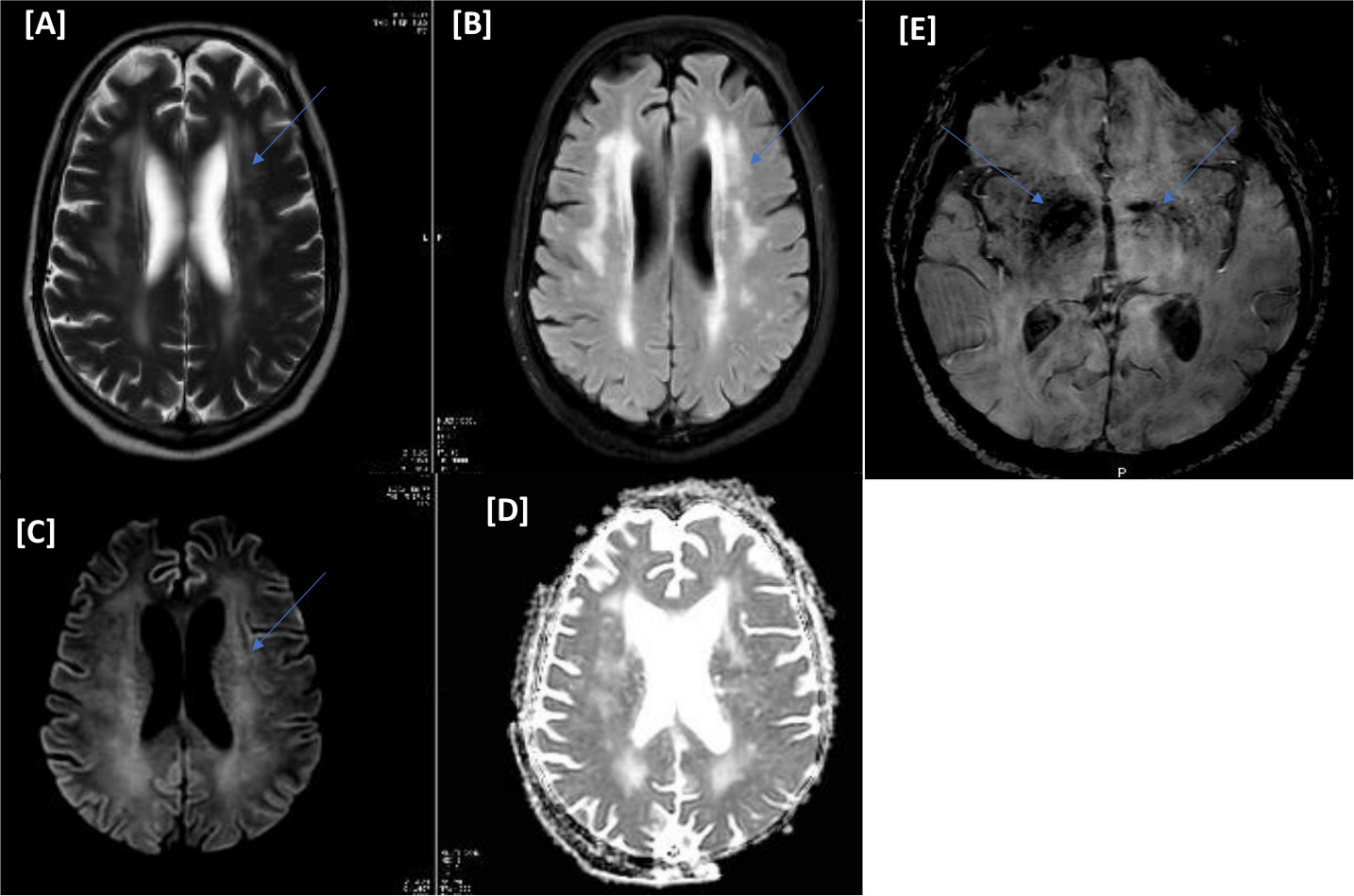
(Case I) [A–E images] Magnetic resonance imaging of the head. Diffuse periventricular white matter hyperintense signals on T2/FLAIR (A and B). No corresponding diffusion restriction on diffusion-weighted imaging b1000/apparent diffusion coefficient (C and D). Bilateral basal ganglia small storm susceptibility appearance on susceptibility-weighted images is more significant on the right side (E).
